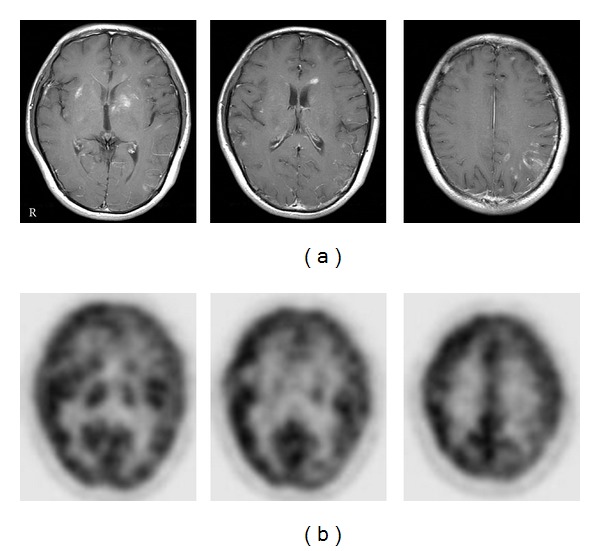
Contrast-enhanced T1-weighted MR (a) and ^18^F-FDG PET (b) images in an HIV-positive patient with toxoplasmosis. MR images show multiple, small, irregular enhanced lesions in the basal ganglia and the white matter. PET images show no ^18^F-FDG uptake in the lesions.
